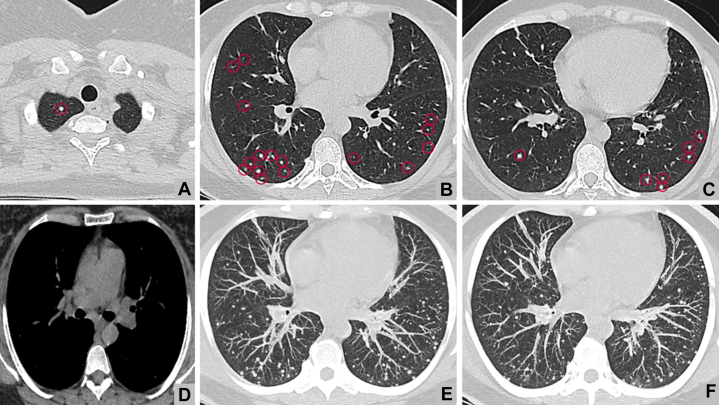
A-F, Initial chest CT scan showing innumerable, randomly distributed bilateral semicalcified pulmonary nodules. A-C, Nodules in various lung regions are demonstrated. D, Borderline mediastinal lymphadenopathy is shown. E-F, Maximum intensity projection reconstructions emphasizing the diffuse and extensive distribution of pulmonary nodules. Selected nodules are marked with crimson circles for clarity.
